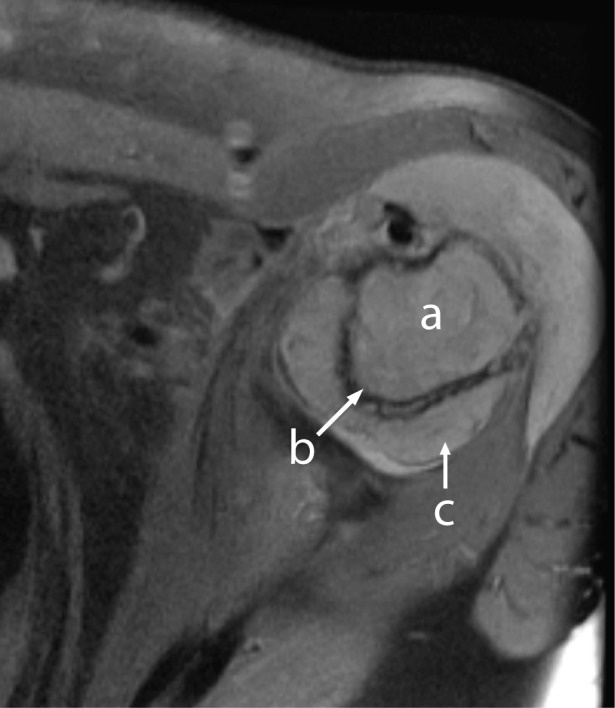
MRI of left shoulder showing abnormal marrow signal of the proximal humerus (a) with cortical erosion (b) and soft tissue mass (c) with joint effusion.
